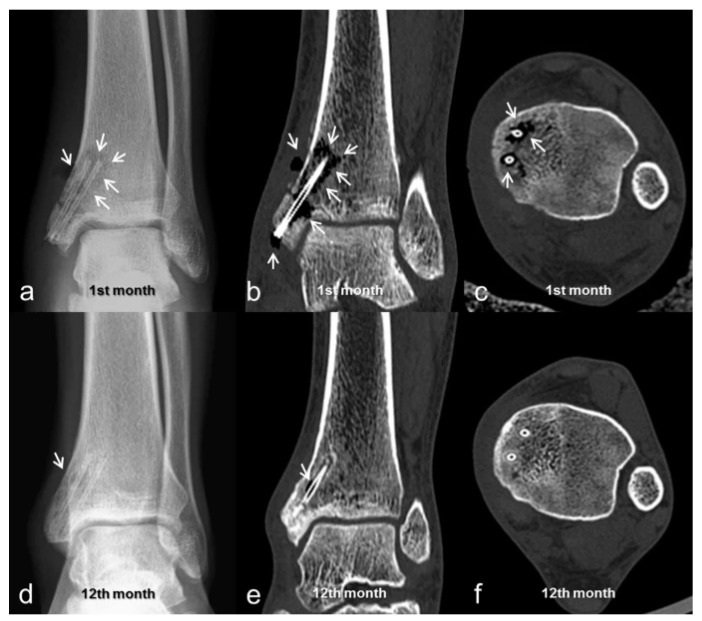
Isolated medial malleolar fracture treated with biodegradable magnesium screws (MAGNEZIX^®^ CS; Syntellix AG, Hanover, Germany). (a–c) taken 1 month after implantation; (d–f) on 12th month after implantation. (a,d) are radiographs; (b,c,e,f) are taken using CT. White arrows point at gas formation around implant. Reproduced from May et al. (2020) [15]. Copyright Springer Nature, 2020.
